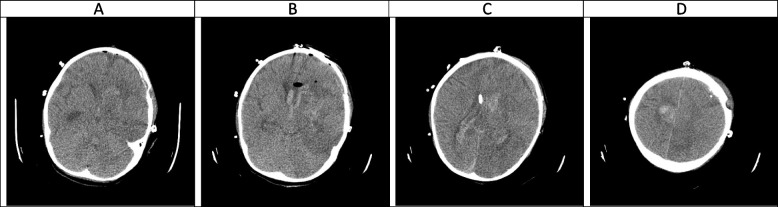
(the brain CT SCAN without contrast, day 7). A-D The most of the post-operation changes including intraventricular and intraparenchymal air as well as parenchymal edema mainly disappeared at this time suggestive of improvement of post-operation changes (only minimal intraparenchymal, intraventricular and extra-axial air bubble is seen). A hyperdense rim seen around the left frontal lobe is suggestive of the remnant of post-operative subdural hematoma. Although some degree of intraventricular hemorrhage still could be seen, but no hydrocephalus is found suggestive of the well-functioning EVD. A remnant of hemorrhagic lesion of high convexity of the right frontoparietal junction is seen. Mentioned hypodense lesions within the cerebellar hemispheres and both cerebral hemispheres are faint in this study which can be due to some degree of improvement
